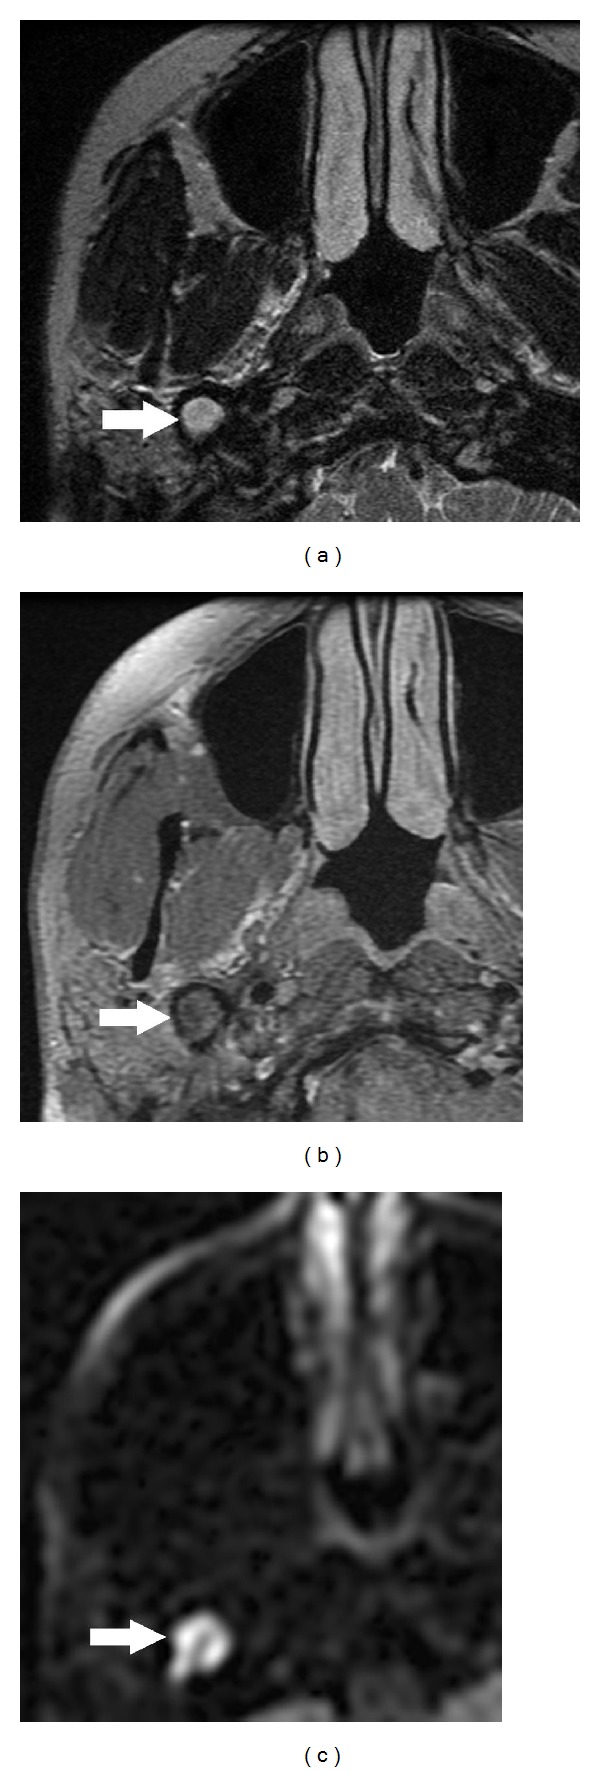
(a) MRI shows that the material in the pneumatised right styloid process is hyperintense on T2-weighted imaging (a), of intermediate signal intensity on fat-suppressed T1-weighted imaging (b), and is markedly hyperintense on PROPELLER (periodically rotated overlapping parallel lines with enhanced reconstruction) diffusion-weighted imaging (c). These signal characteristics are typical of cholesteatoma.
